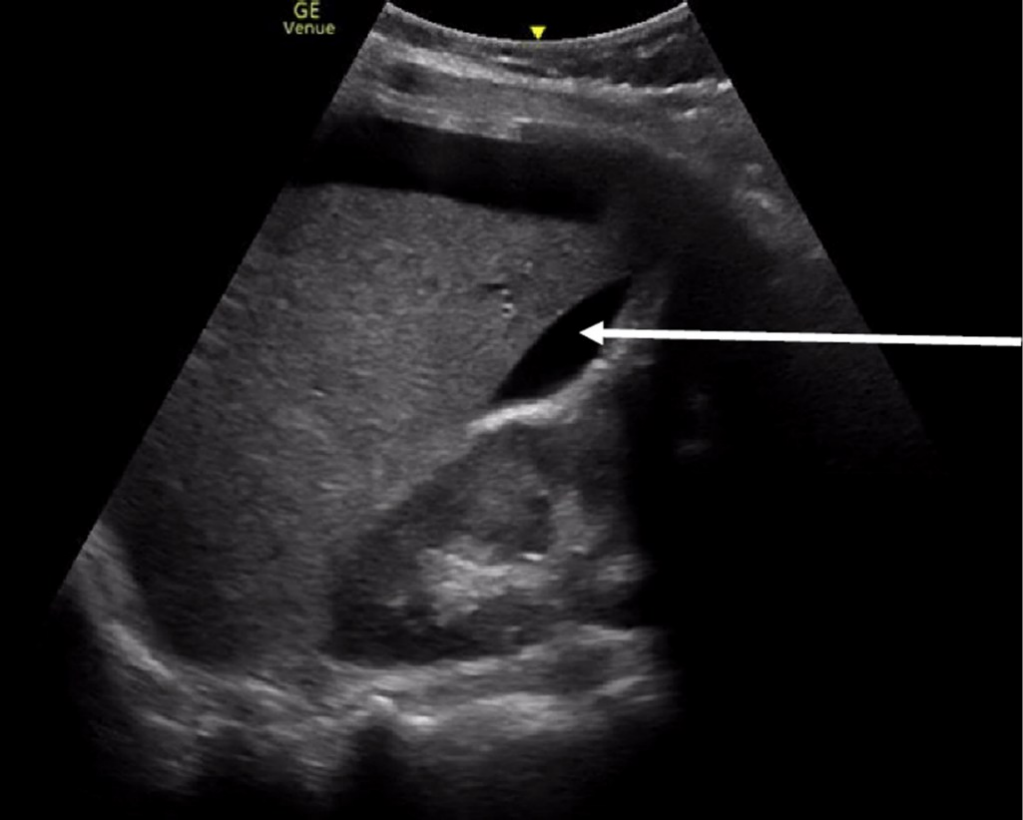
Point of care ultrasound (POCUS) demonstrating anechoic free fluid in the right upper quadrant along the hepatorenal space (white arrow) consistent with a ruptured ectopic pregnancy.
